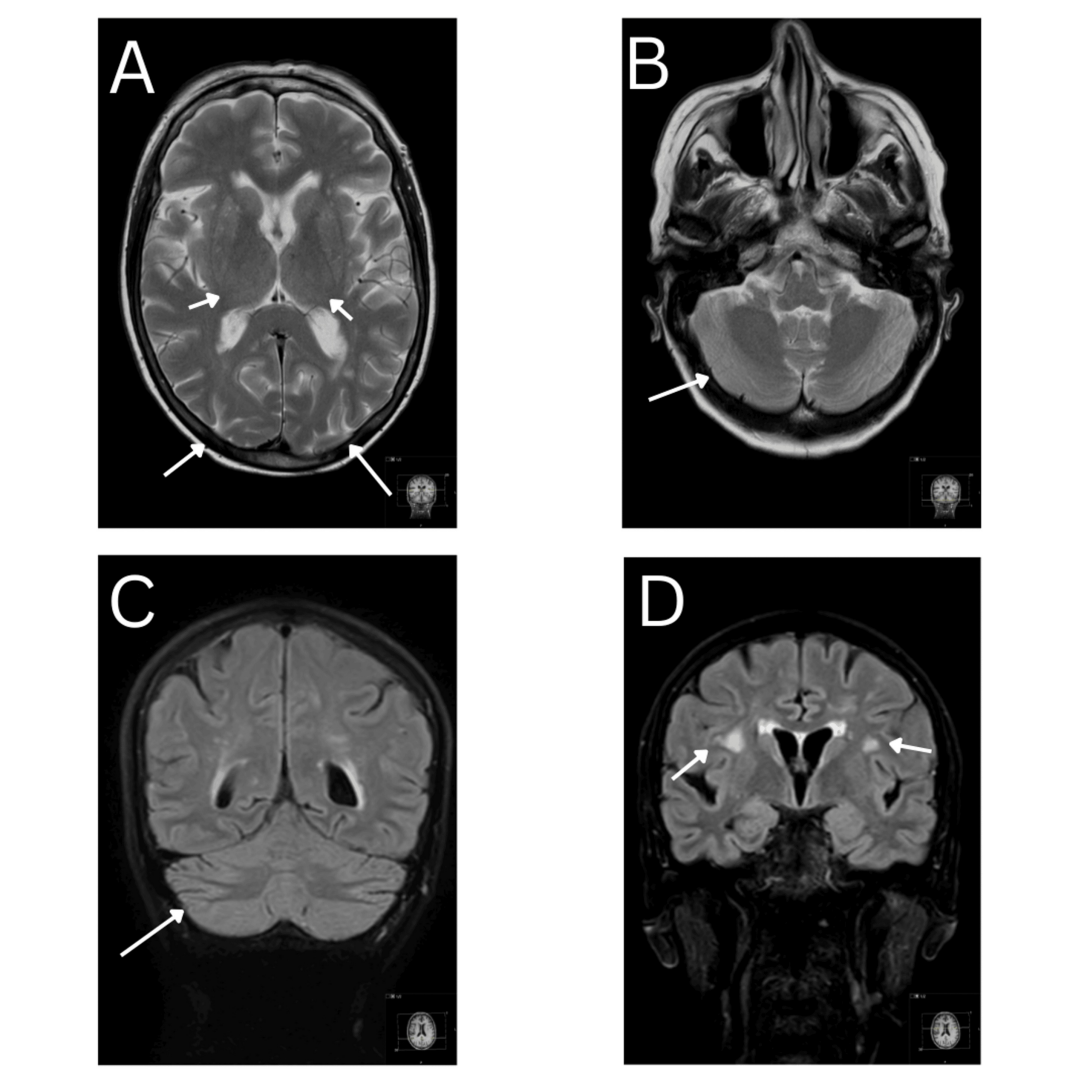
Resolution of changes in cerebrum and cerebellum confirming posterior reversible encephalopathy syndrome (PRES) A: Axial MRI head showing resolution of signal changes within the subcortical white matter of basal ganglia, both occipital lobes and thalami B: Axial MRI head showing resolution of signal changes in cerebellum C: Coronal MRI head showing resolution of changes in cerebellum on fluid-attenuated inversion recovery (FLAIR) MRI D: Coronal MRI head showing resolution of changes in inferior temporal lobe and basal ganglia on FLAIR MRI
